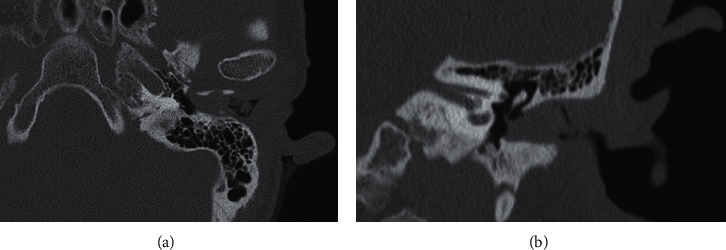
Temporal bone computed tomography revealing a 0.5 × 0.5 cm low-density mass in the cartilaginous portion of the left external auditory canal and low-density material with adjacent bony erosion medial to the mass: (a) axial view; (b) coronal view.
